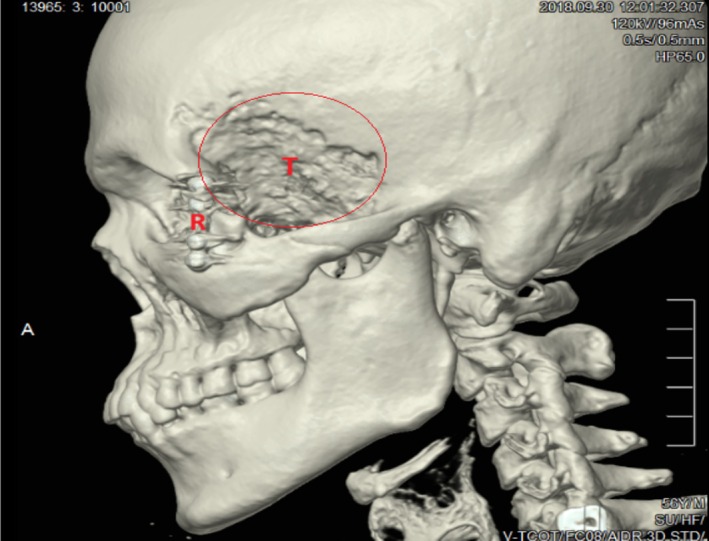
Computed tomography (CT) scan of the facial skeleton showing (R) the internal fixation at the lateral rim of the left bony orbit and (T) left fronto‐temporal lytic lesions.
